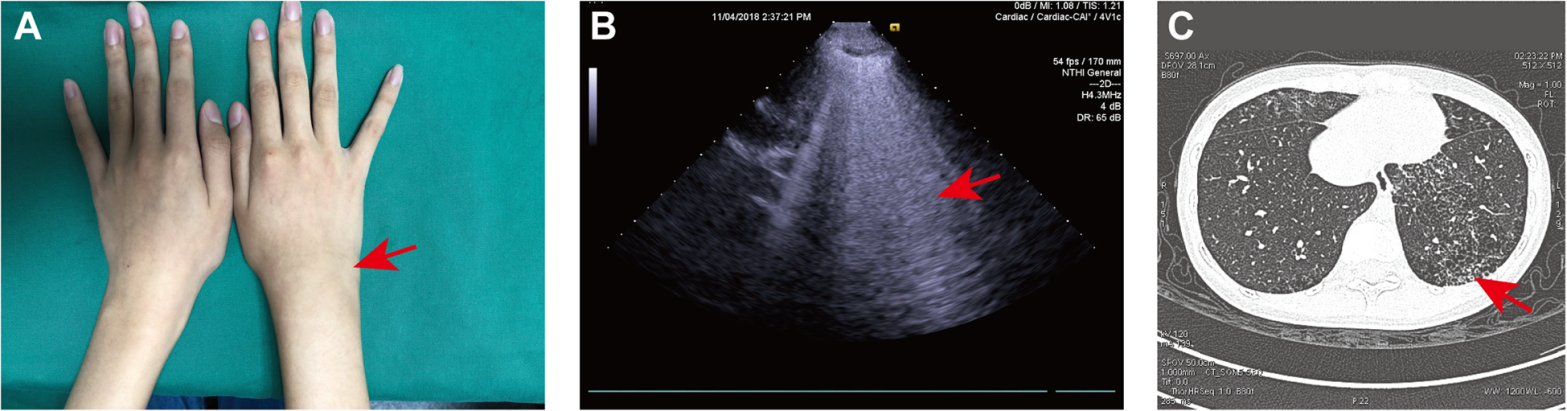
Case 1. A Physical examination showed right wrist swelling (arrow). B Lung ultrasound revealed multiple B-lines (arrow). C HRCT demonstrated diffuse ground glass opacity, reticular abnormalities, and traction bronchiectasis (arrow)
